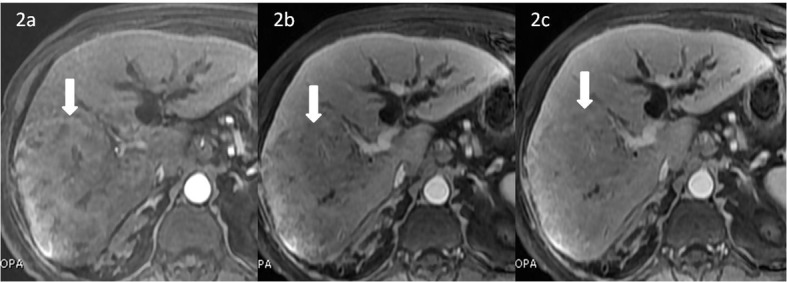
Dynamic contrast-enhanced MRI (CEMRI) T1-weighted fat suppressed sequence using hepatocyte specific contrast gadobenate dimeglumine (Gd BOPTA) showing lesion enhancement pattern. (a) Lesion in the right lobe (white bold arrow pointing down) shows mild enhancement in the hepatic arterial phase (HAP). (b) Lesion appears relatively hypointense with better visualization of the lesion (white bold arrow pointing down) on the portal venous phase (PVP). (c) Lesion appears iso-hypointense compared to rest of the liver parenchyma (white bold arrow pointing down) on the equilibrium phase.
